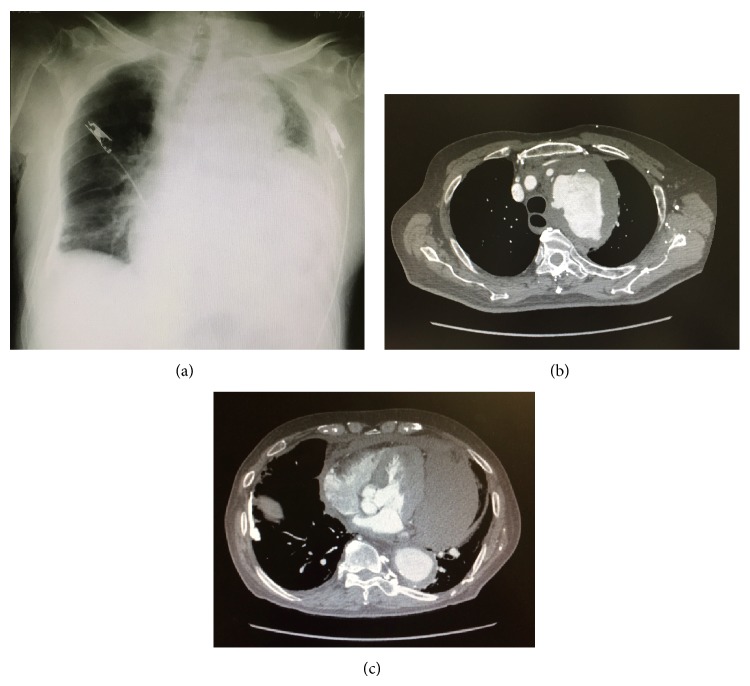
(a) A plain radiograph of the patient's chest revealed a massive left pleural effusion. (b) and (c) Contrast-enhanced computed tomography revealed the presence of an aortic arch aneurysm surrounded by a hematoma, as well as extravasation of contrast media. The hematoma spread from around the aorta towards the mediastinum.
